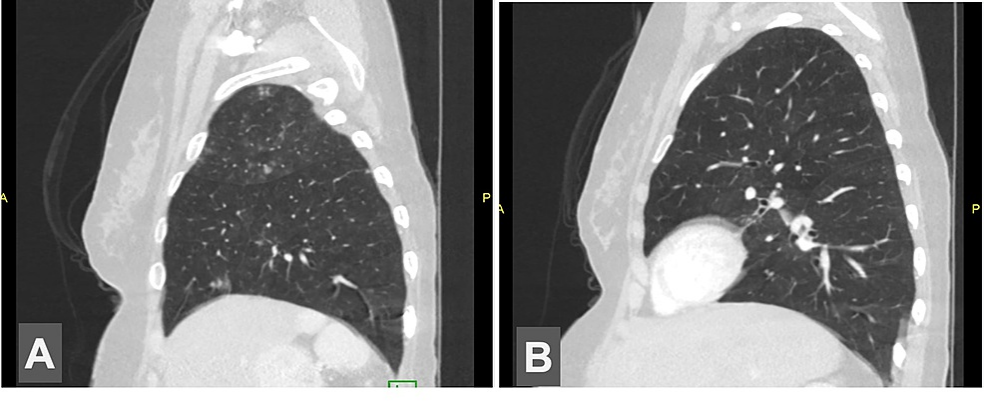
Lateral abdomen CT images A. Bilobar right lung. B. Bilobar left lung CT: computed tomography
